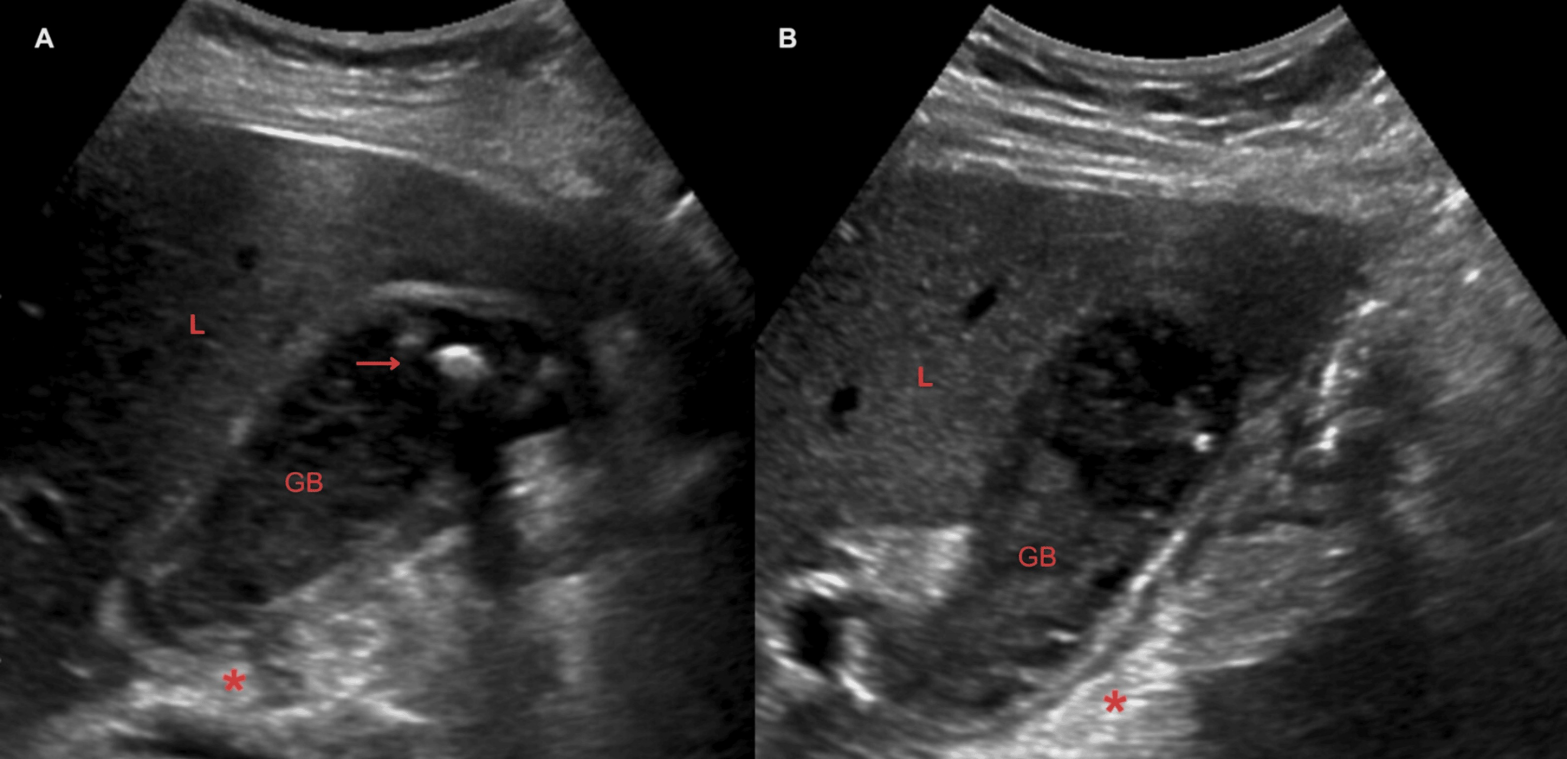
Abdominal ultrasound The arrow shows a rounded echogenic image. The asterisk (*) shows edema and perivesicular fluid. A: GB with lithiasis. B: GB and Hartmann pouch. GB: gallbladder, L: liver
